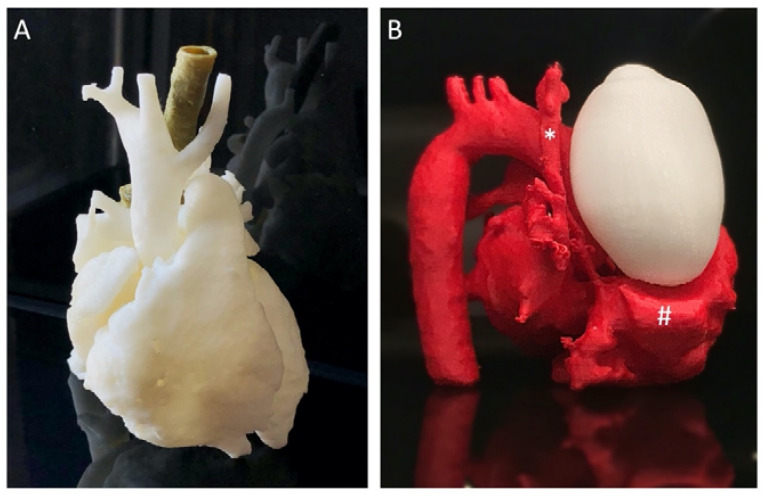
Examples of multi-part models. (A) a case of aortic ring, to visualize a compressed trachea by adjacent, abnormally positioned vessels, a cardiovascular model is printed in white and, separately segmented, trachea is printed green and fitted in place; (B) a case of neonate with a mediastinal tumor (white) compressing the cardiovascular structures (red), the superior vena cava (asterisk) and the right atrium (hashtag), the model was based on multimodality imaging owing to the fact that Angio CT has superior special and temporal resolution for cardiovascular structures while MRI has superior ability to differentiate soft tissue structures and tumors. Parts of the models coming from different modalities were assembled in post-production.
